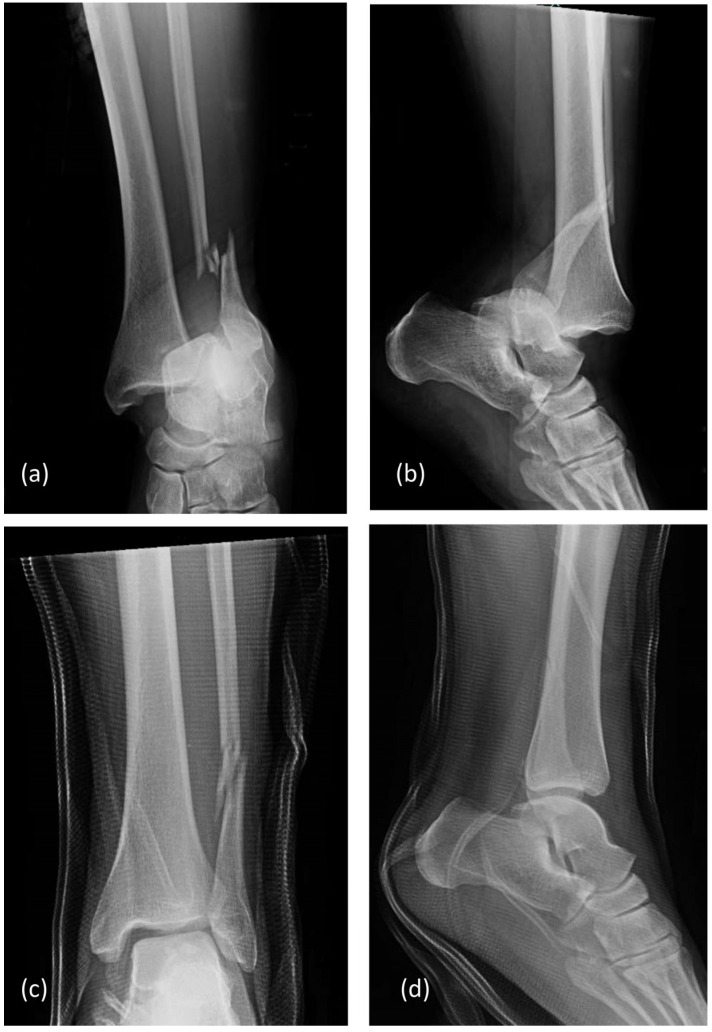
Injury radiographs of left ankle AP (a) and lateral (b) views showing posterolateral fracture dislocation. Post-reduction radiographs AP (c) and lateral (d) views showing restoration of gross alignment.
